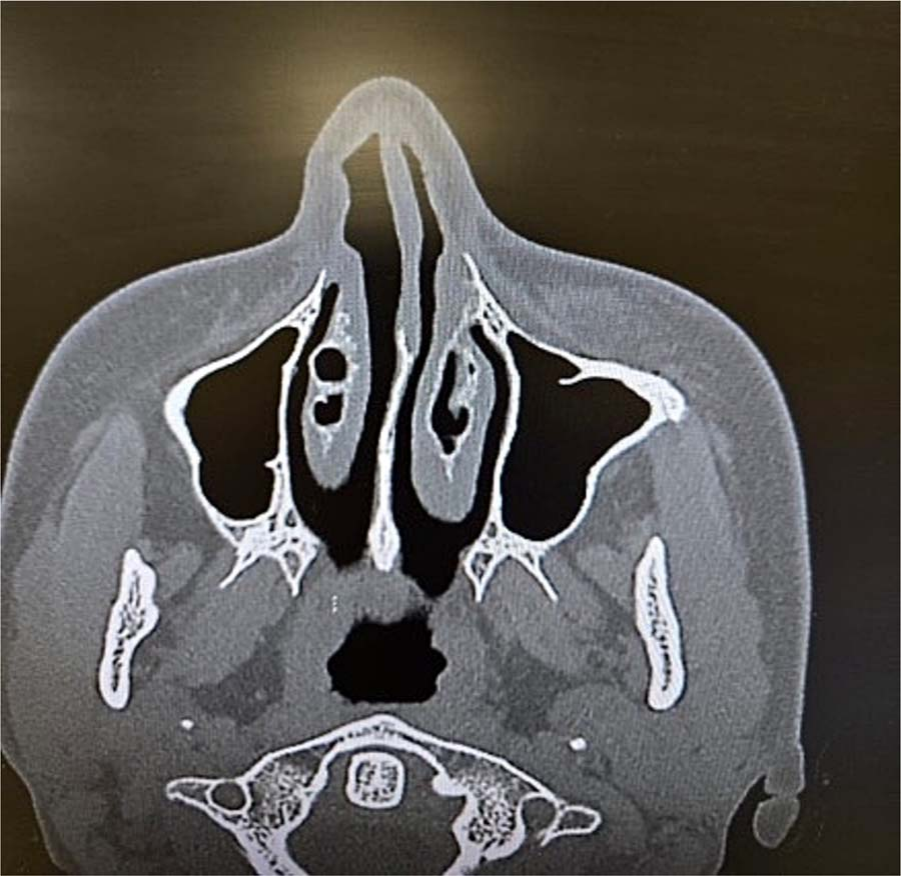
Paranasal sinus CT scan in the axial plane demonstrating inferior concha bullosa.
